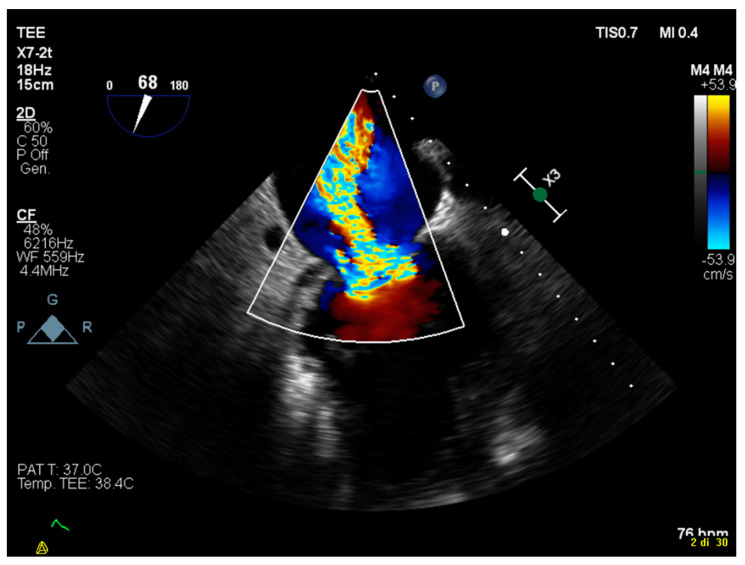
An intercommissural view on the transesophageal echocardiogram highlights severe mitral insufficiency with prevalent mitral regurgitation jet from the A2-P2 mitral valve scallops.
